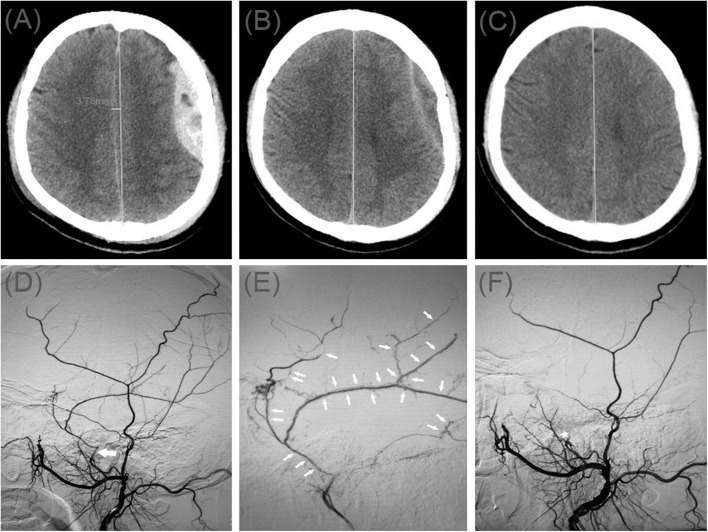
(A) Brain computed tomographic hematoma images for patient 2 before treatment (midline shift: 3.78 mm). (B) Brain CT was repeated on postoperative day 10. (C) Follow-up brain CT was performed at 67 days. (D) Pre-operative DSA presented abnormal MMA (Large arrow). (E) Intra-operative DSA showed contrast agent extravasation or arteriovenous fistula (Small arrow). (F) Postoperative DSA found that the MMA disappeared (Asterisk).
